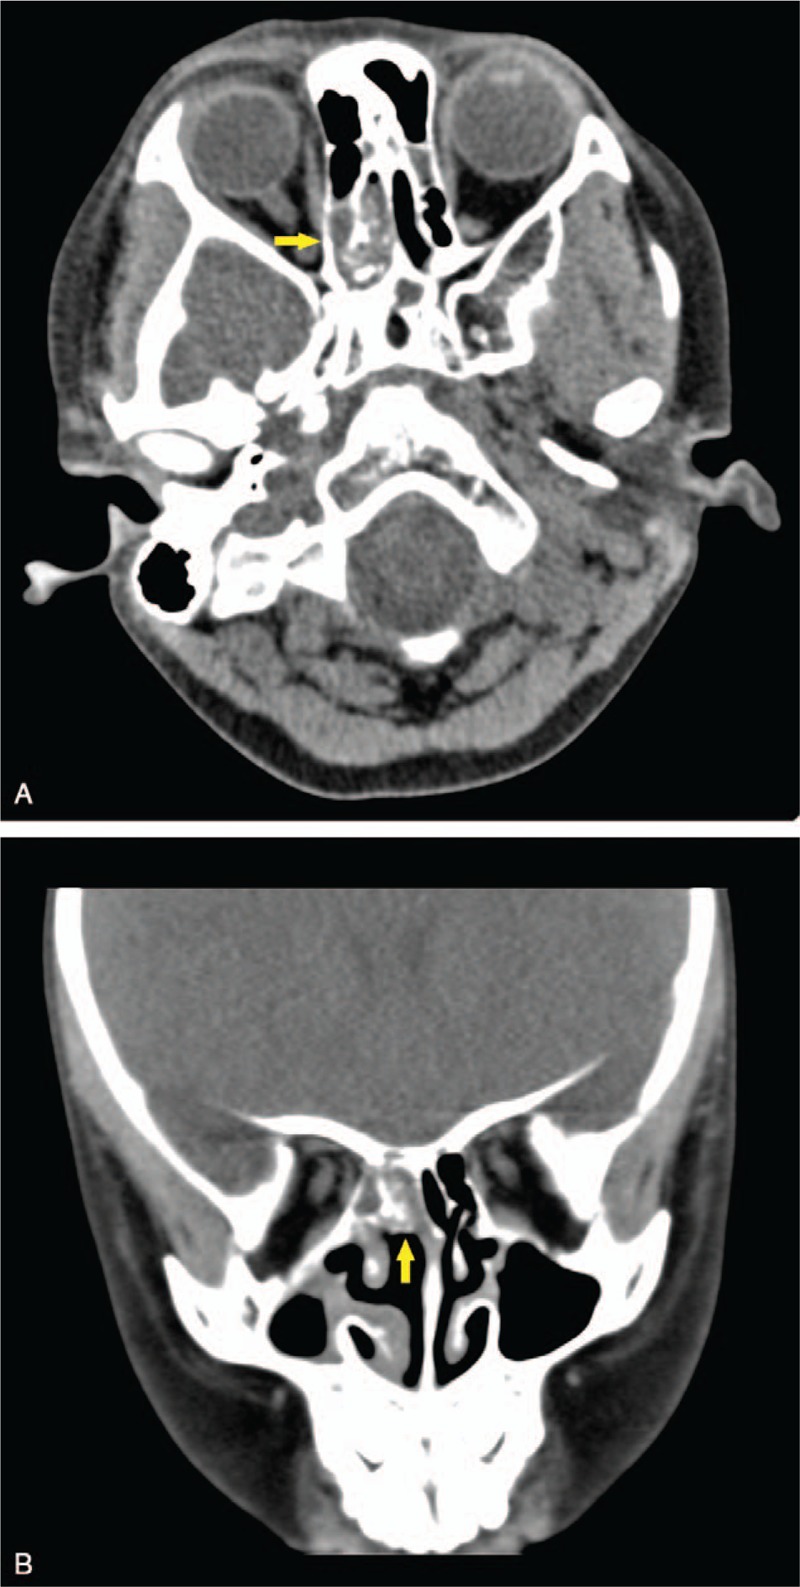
Computed tomography revealed soft tissue density mixed with multiple discrete hyperdense calcification in the right posterior ethmoid sinus (yellow arrow).
